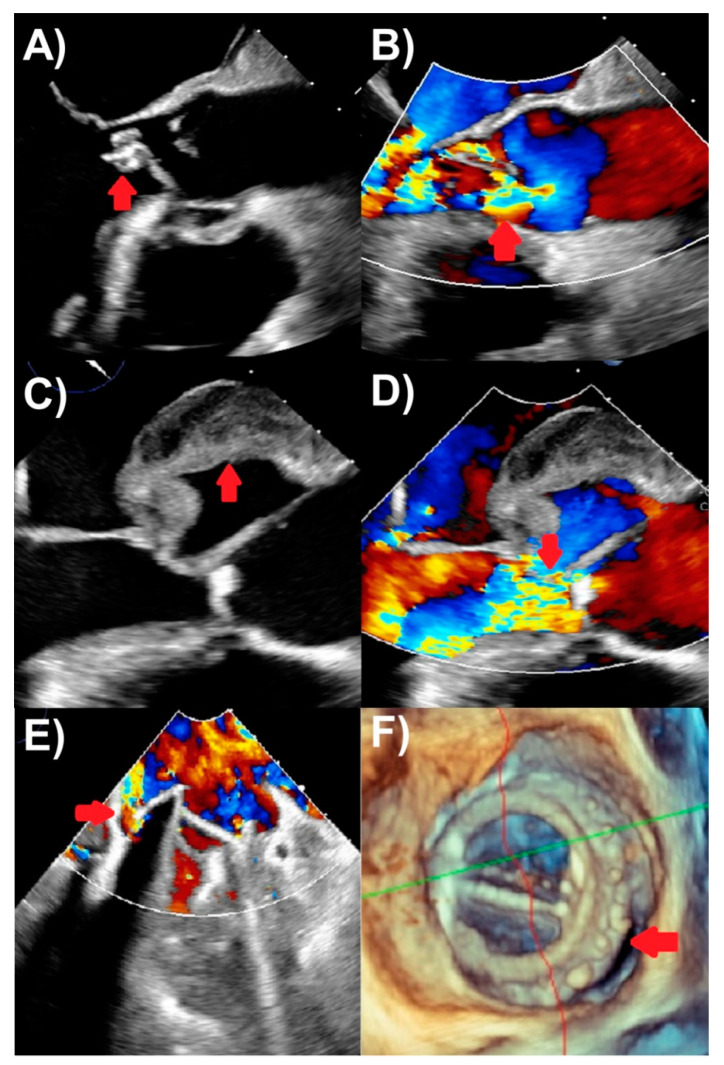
Transesophageal echocardiography findings of endocarditis. (A) Aortic valve vegetation (arrow), with (B) severe aortic regurgitation (arrow) on color Doppler. (C) Aortic with echolucent space consistent with pseudoaneurysm and abscess (arrow), with (D) severe aortic regurgitation (arrow) on color Doppler. (E) Mechanical mitral valve replacement paravalvular regurgitation (arrow) associated with (F) prosthetic valve dehiscence (arrow) seen on three-dimensional assessment.
